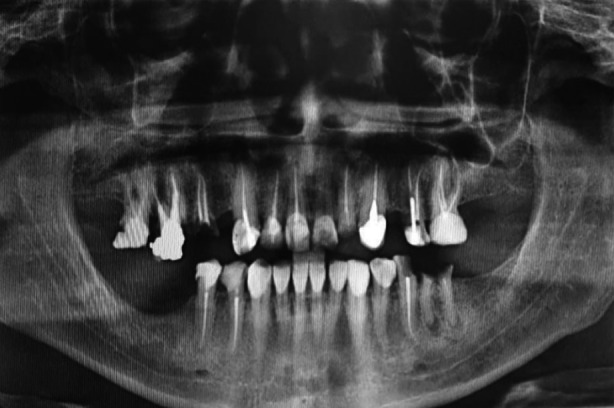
Postoperative Panoramic radiograph showed complete removal of sialolith.
